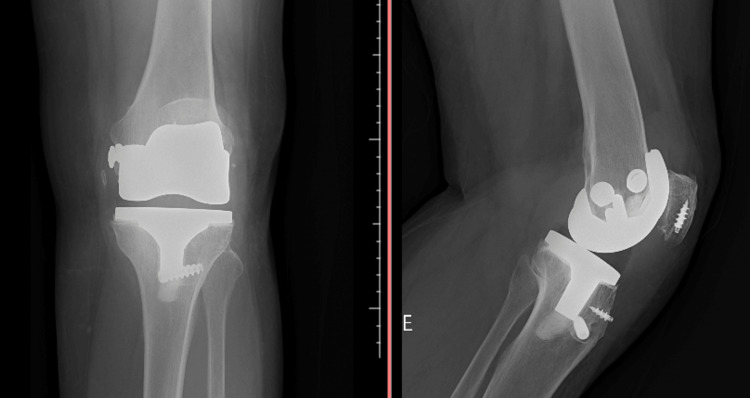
﻿﻿Knee radiograph in AP and lateral views, showing adequate patellar positioning. AP: anteroposterior
